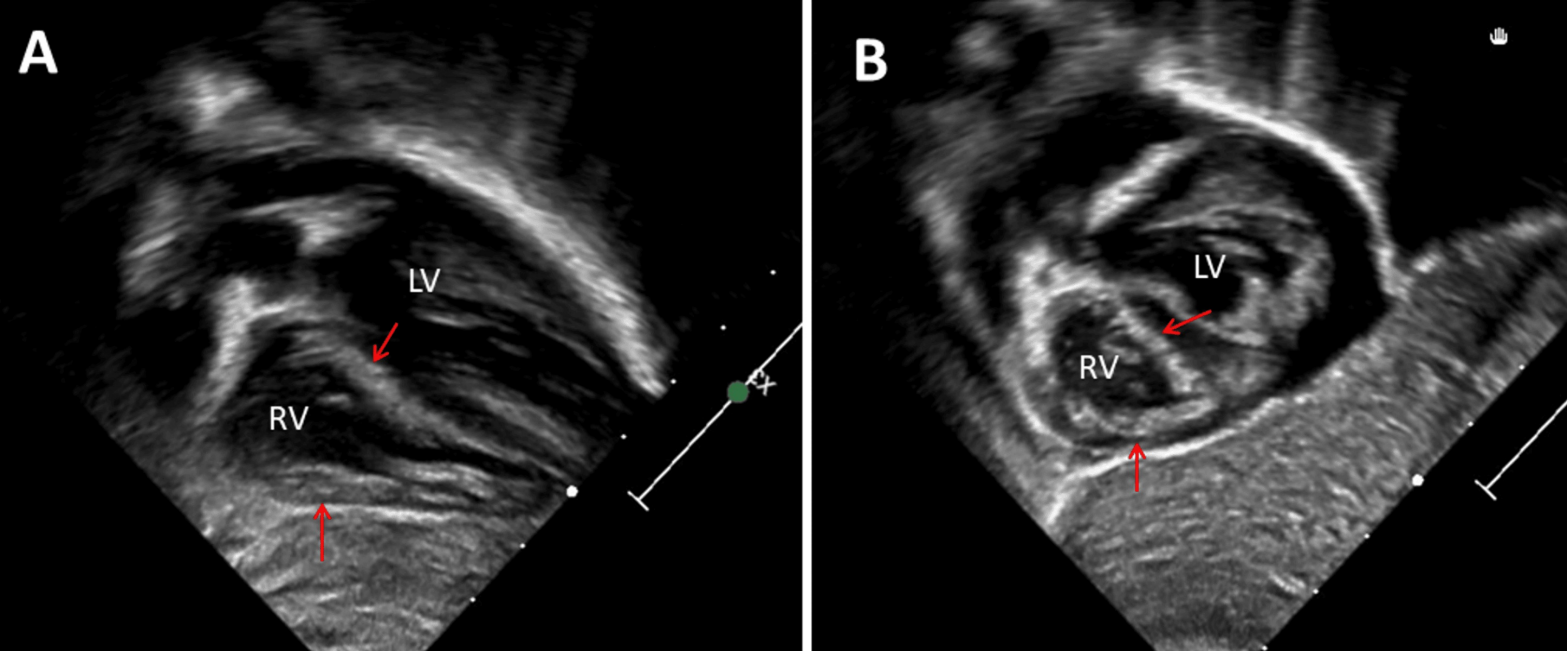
Transthoracic echocardiography showing right ventricular hypertrophy, which has not been found on previous examinations, and mild enlargement of the right ventricle (panels A and B).
